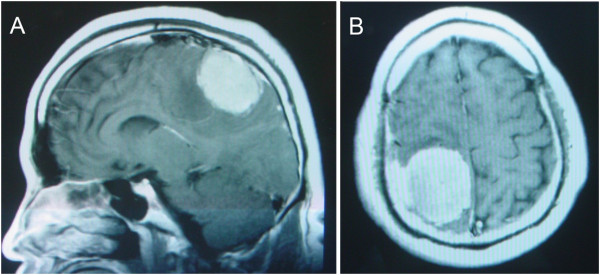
Images of the patient before the first procedure. Sagittal (A) and axial (B) contrast-enhanced MRI scan prior to the first admission demonstrating an enhanced lesion located in the right parietal lobes with the enhanced meninges. (T1W).
